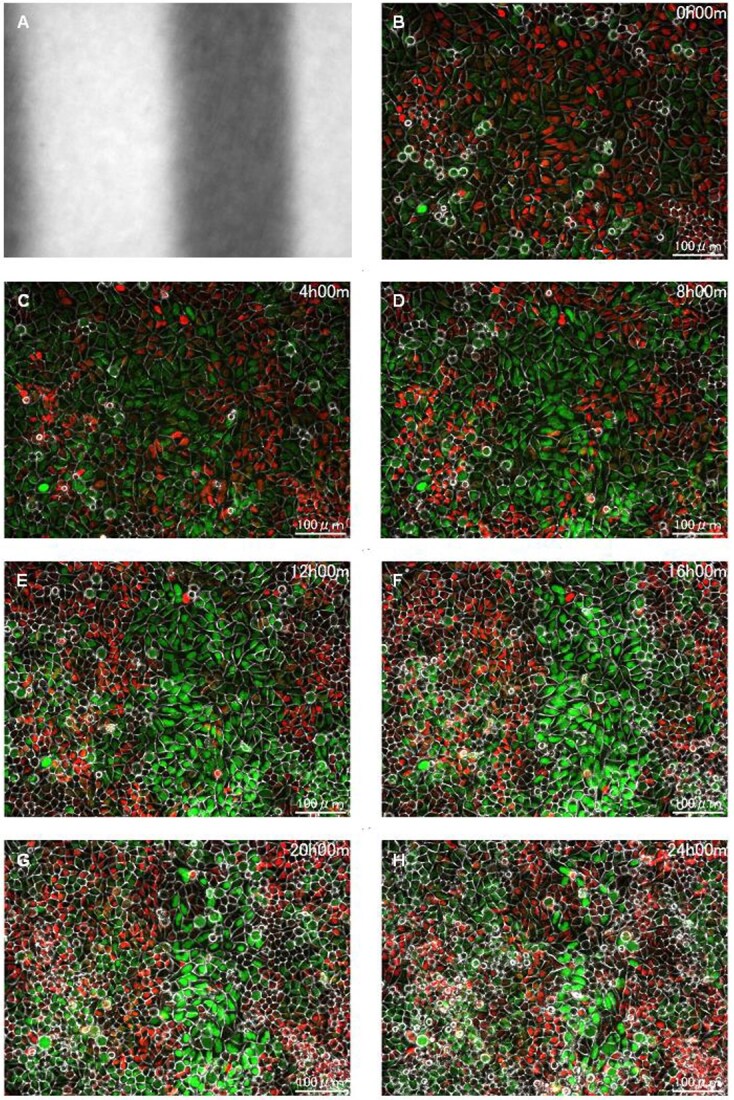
Magnified time-lapse imaging on HeLa-FUCCI cells following exposure to spatially fractionated X-rays. (A) Dose profiles of the 300-μm-slit field using a Gafchromic XR-RV3 radiochromic film. (B–H) Representative magnified fluorescent images of HeLa-FUCCI cells observed 20× magnification objective lens at 0 (B), 4 (C), 8 (D), 12 (E), 16 (F), 20 (G) and 24 h following exposure to the 300-μm-slit X-rays (H). Cell nuclei are indicated by the red, yellow, green and colorless regions depending on their cell-cycle phase, G1, G1/S, S/G2 and M, respectively. Scale bar—100 μm.
